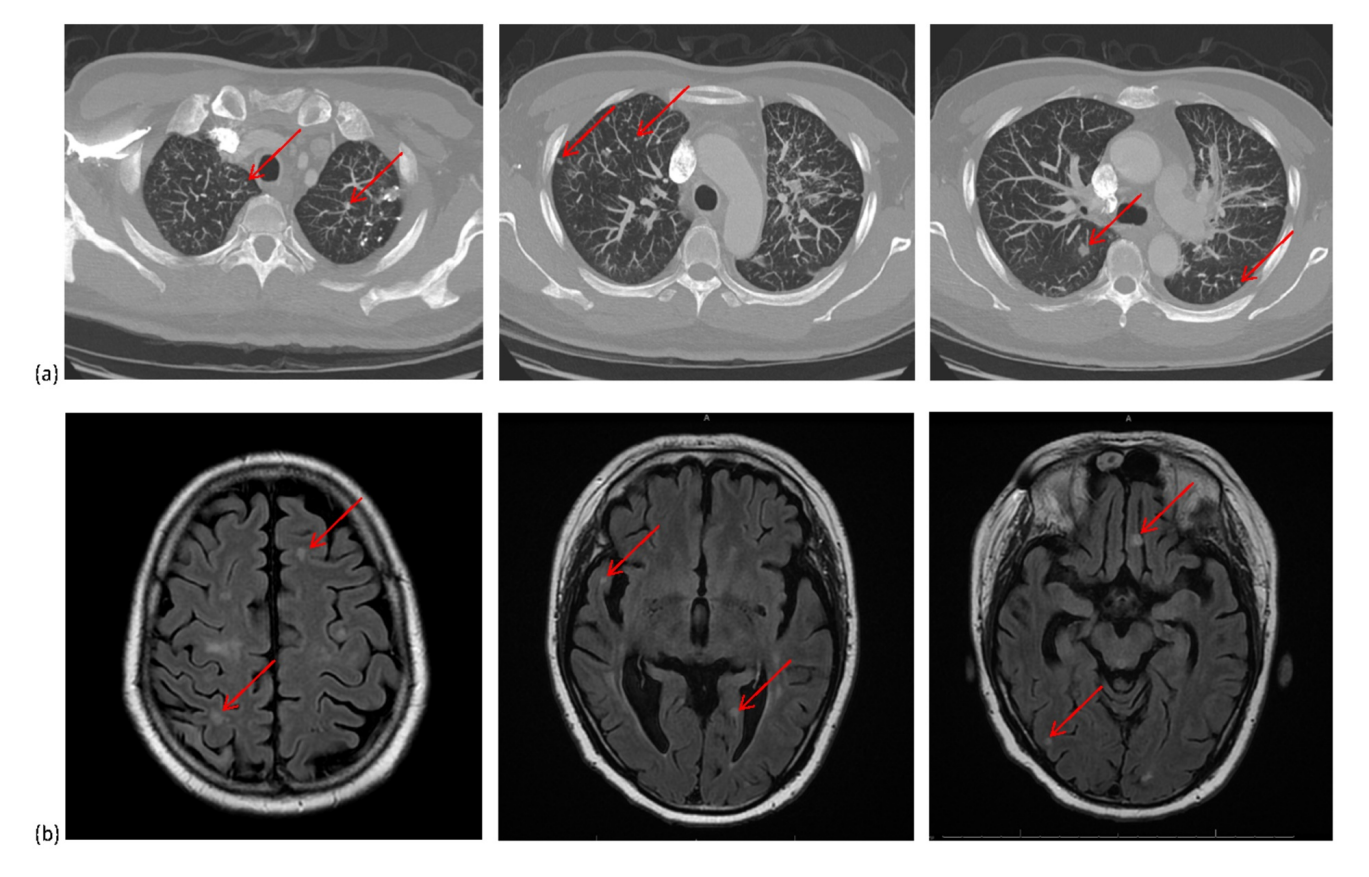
(a) Axial computed tomography (CT) images of the chest and (b) axial magnetic resonance imaging (MRI) of the brain showing tiny, diffuse, nodular metastases
